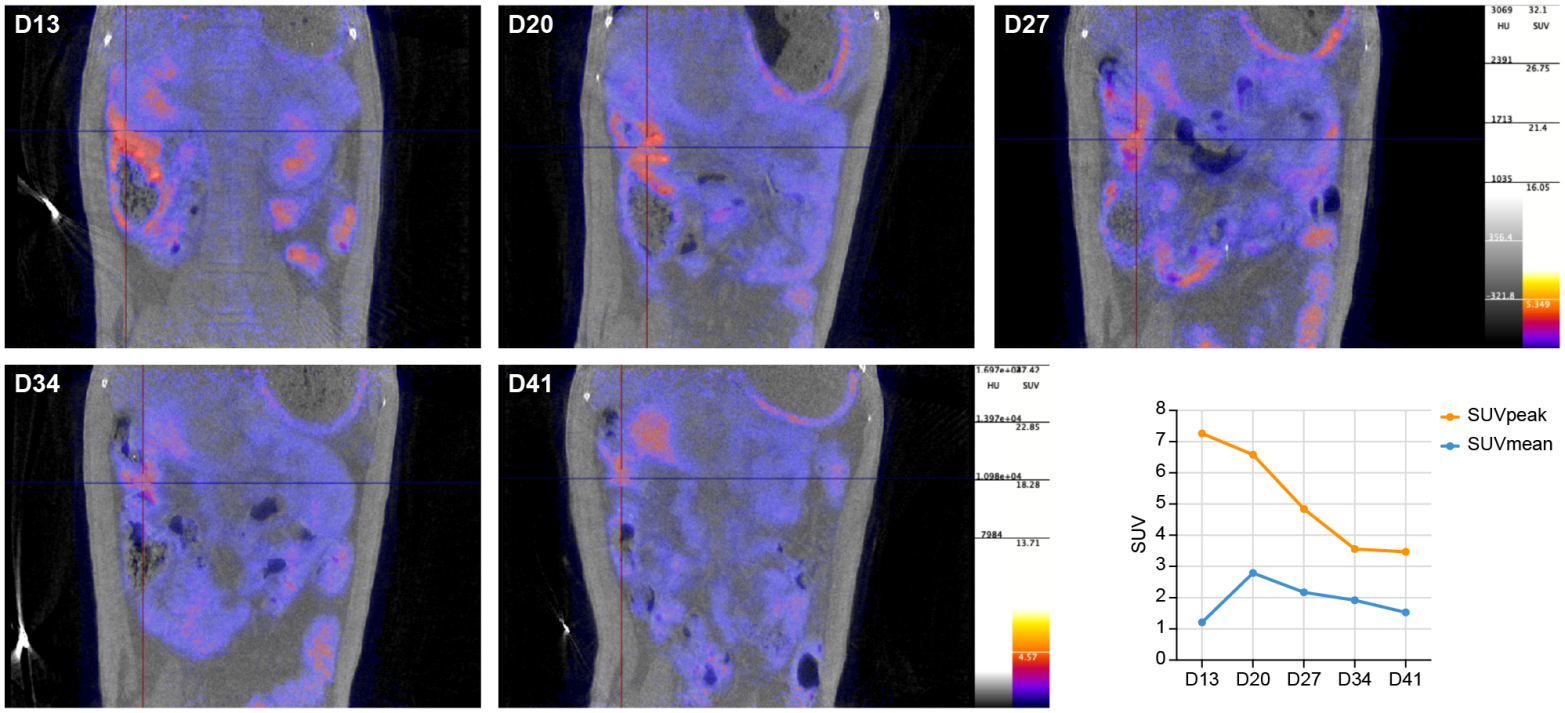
Longitudinal development of metabolic activity by increased [^18^F]FDG uptake in the ileum from day 13 to day 41 post infection. The ileum is marked by the crosshair. The graph (bottom, right) shows the calculated SUVs throughout the study.
